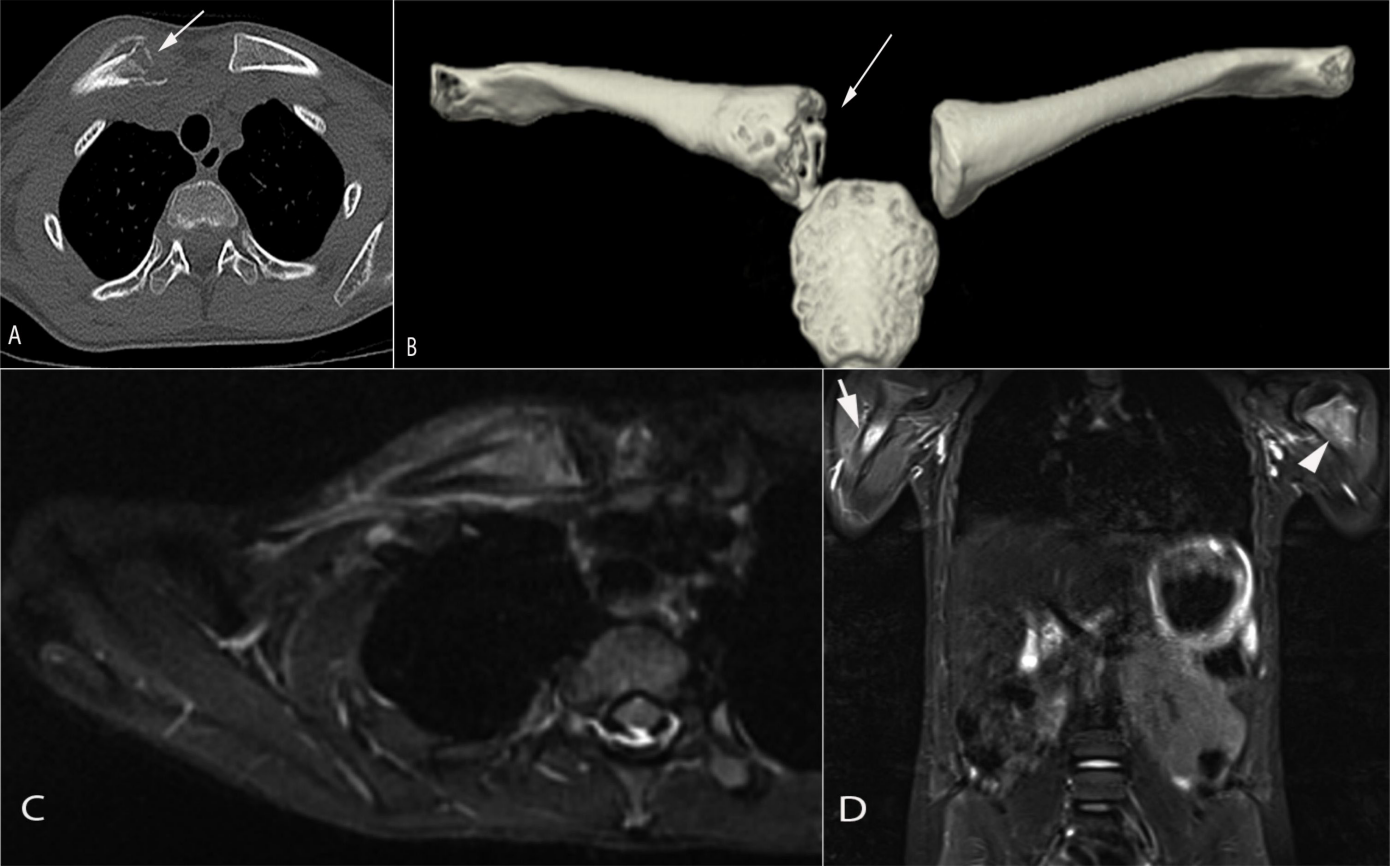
An 8-year-old girl presented with a painful lump on the right clavicle. Axial (A) and 3D reformats (B) computed tomography images demonstrate a destructive lesion in the medial aspect of the right clavicle associated with periosteal reaction and soft tissue swelling (arrow in a/b). Axial T2 STIR magnetic resonance image (C) showed a marked signal abnormality involving the medial aspect of the right clavicle with associated periosteal fluid and soft tissue edema. Total body MRI-STIR sequences (D) showed additional lesions in the proximal right (arrow) and left (arrowhead) humeral metaphysis, right iliac crest (not shown)and left greater trochanter (not shown). Findings are consistent with CRMO following a Whole Body MRI.
